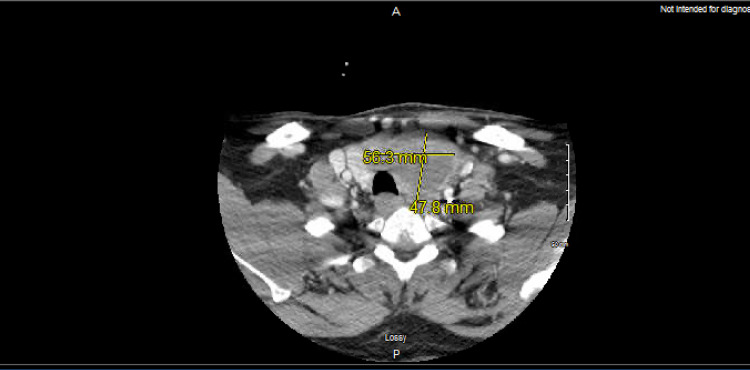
CT neck with contrast showing the thyroid mass CT: computed tomography
